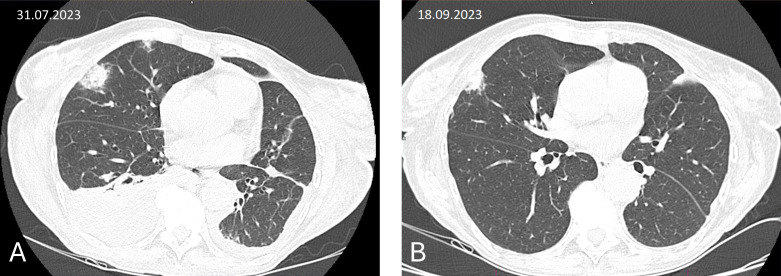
Thorax CT examination showing suspicious right lung focal lesions and bilateral pleural effusion, manifested more severely on the right side (A). Follow-up thorax CT presenting with partial response of the observed right lung lesions (reduction in size) and complete resolution of pleural effusion despite the discontinuation of ICI therapy (B).
